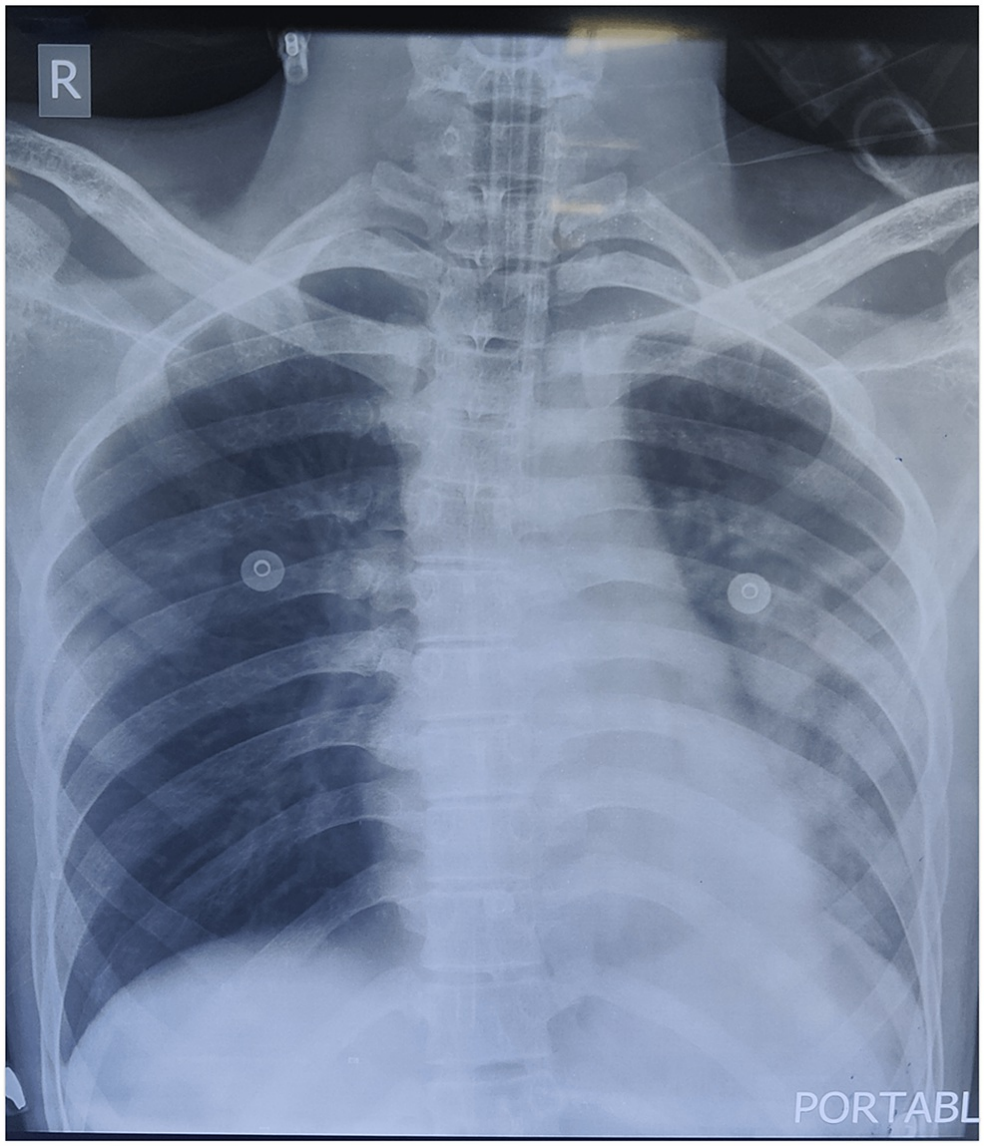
Portable X-ray chest of the male patient with homogenous opacities visualized in the left lung involving the lower lobe predominantly
